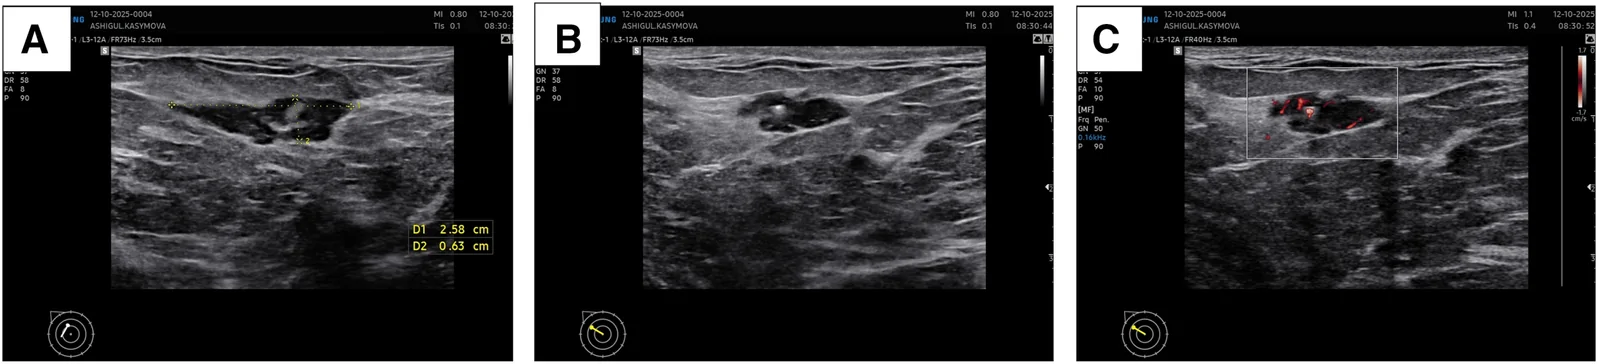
Follow-up ultrasonographic imaging findings after completion of the first treatment cycle. (A) Ultrasonography showed a hypoechoic mass measuring approximately 25.8 × 6.3 × 14 mm at the 11-o’clock position of the right breast. (B) The mass had relatively well-defined margins and an irregular shape, with internal septation-like echoes and punctate hyperechoic foci. (C) Color Doppler flow imaging demonstrated linear blood flow signals within the lesion.
